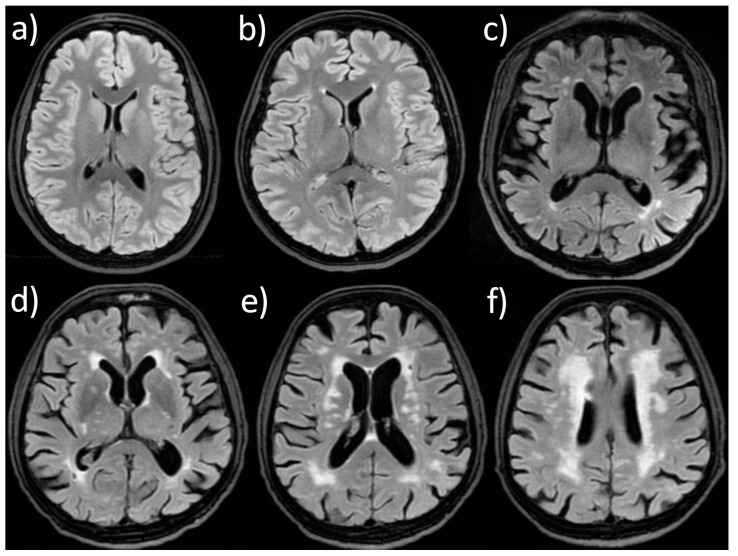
FLAIR images showing (a) the absence of LA; (b) caps on the frontal horns and a single punctate subcortical frontal focus—Fazekas 1; (c) caps around the occipital horns with several foci—Fazekas 1; (d) halo around the frontal and occipital horns—Fazekas 2; and (e,f) confluent periventricular and deep white matter LA in the same patient—Fazekas 3.
